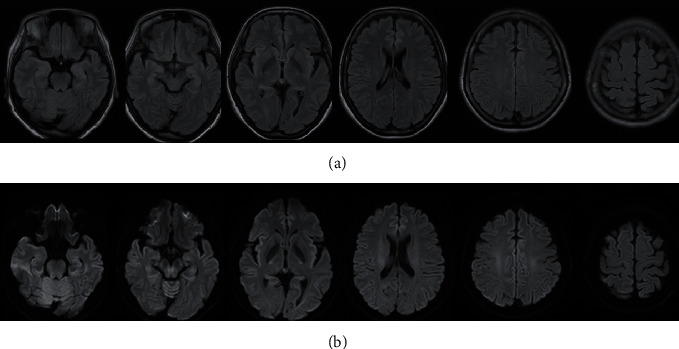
Cranial magnetic resonance imaging findings. Fluid-attenuated inversion recovery imaging (a) and diffusion-weighted imaging (b) at 2 months before treatment.
